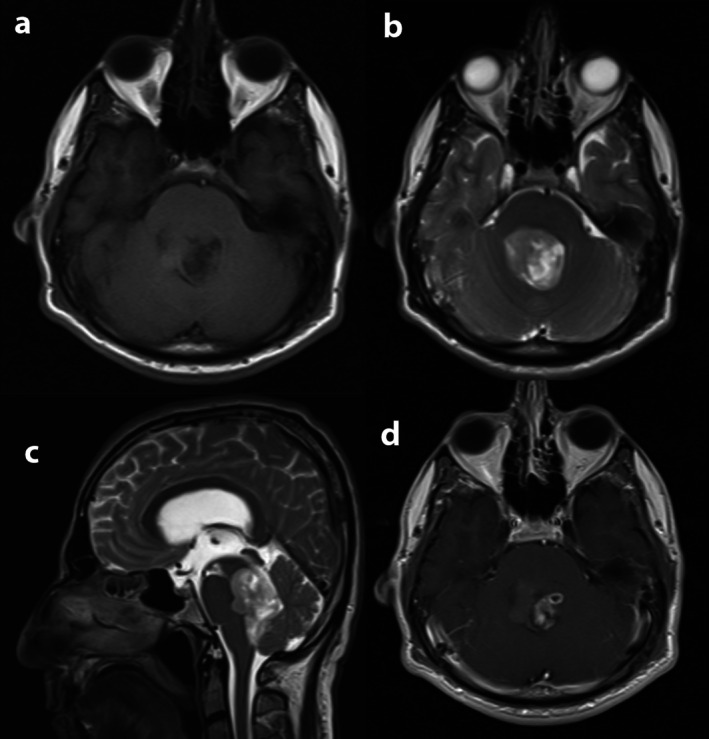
Preoperative MRI: (a) Axial T1W. (b) Axial T2W. (c) Sagittal T2W. (d) T1W with gadolinium.
